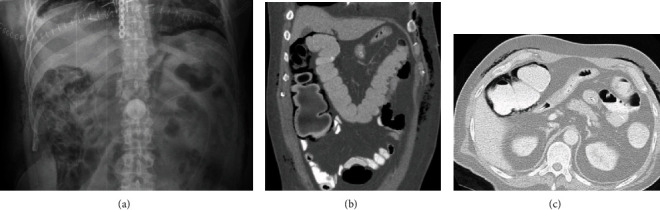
(a) Abdominal radiograph, (b) coronal CT, and (c) axial CT. Pneumatosis intestinalis of the ascending and proximal transverse colon displaying circumferential bubbly/expansile appearance of the colon wall. Notice also the presence of subcutaneous emphysema.
